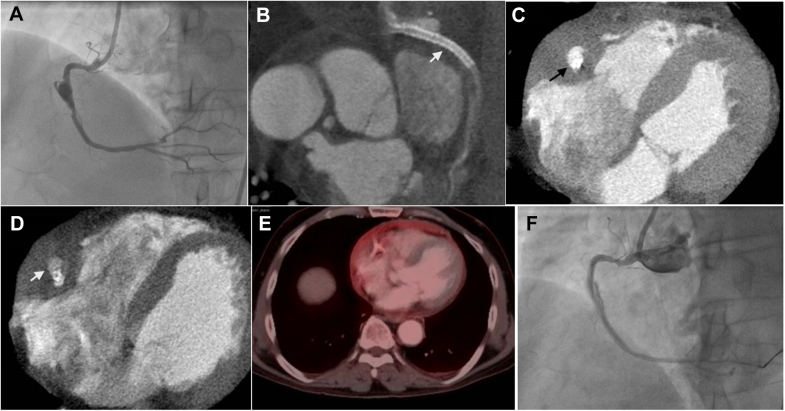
Case 3: Right Coronary Artery Pseudoaneurysm Treated With Covered Stent Implantation (A) Repeat coronary angiography after fever onset showing pseudoaneurysm arising from the stented right coronary artery segment. (B to D) Contrast-enhanced computed tomography images demonstrating the right coronary artery pseudoaneurysm (white and black arrows). (E) Fluorodeoxyglucose positron emission tomography-computed tomography showing no abnormal uptake at the pseudoaneurysm site. (F) Final angiogram after covered stent implantation showing exclusion of contrast filling into the pseudoaneurysm.
